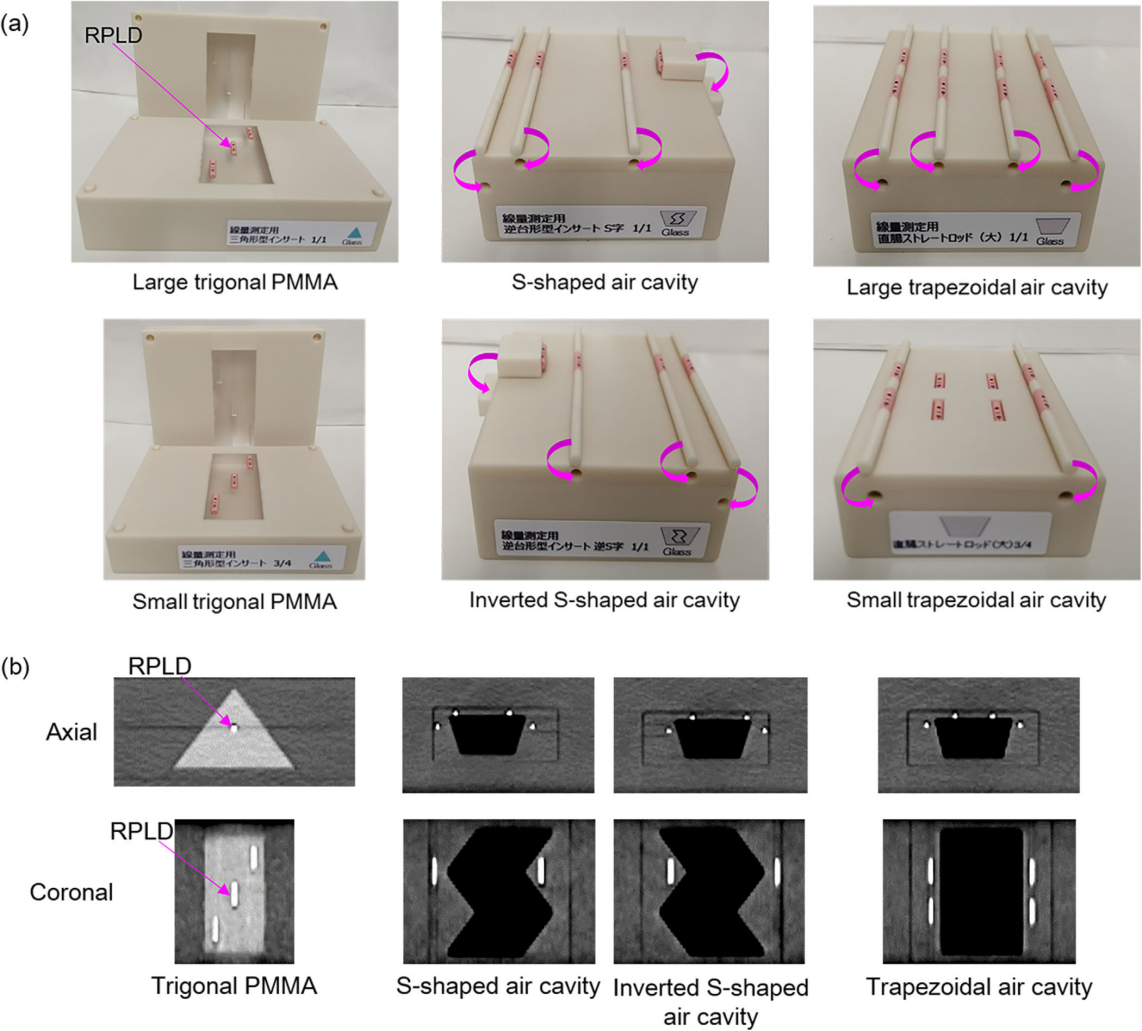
Overview of new custom insert with glass dosimeter. (a) Tumor‐proxy and rectum‐proxy custom inserts. (b) computed tomography (CT) images of tumor‐proxy and rectum‐proxy custom inserts
